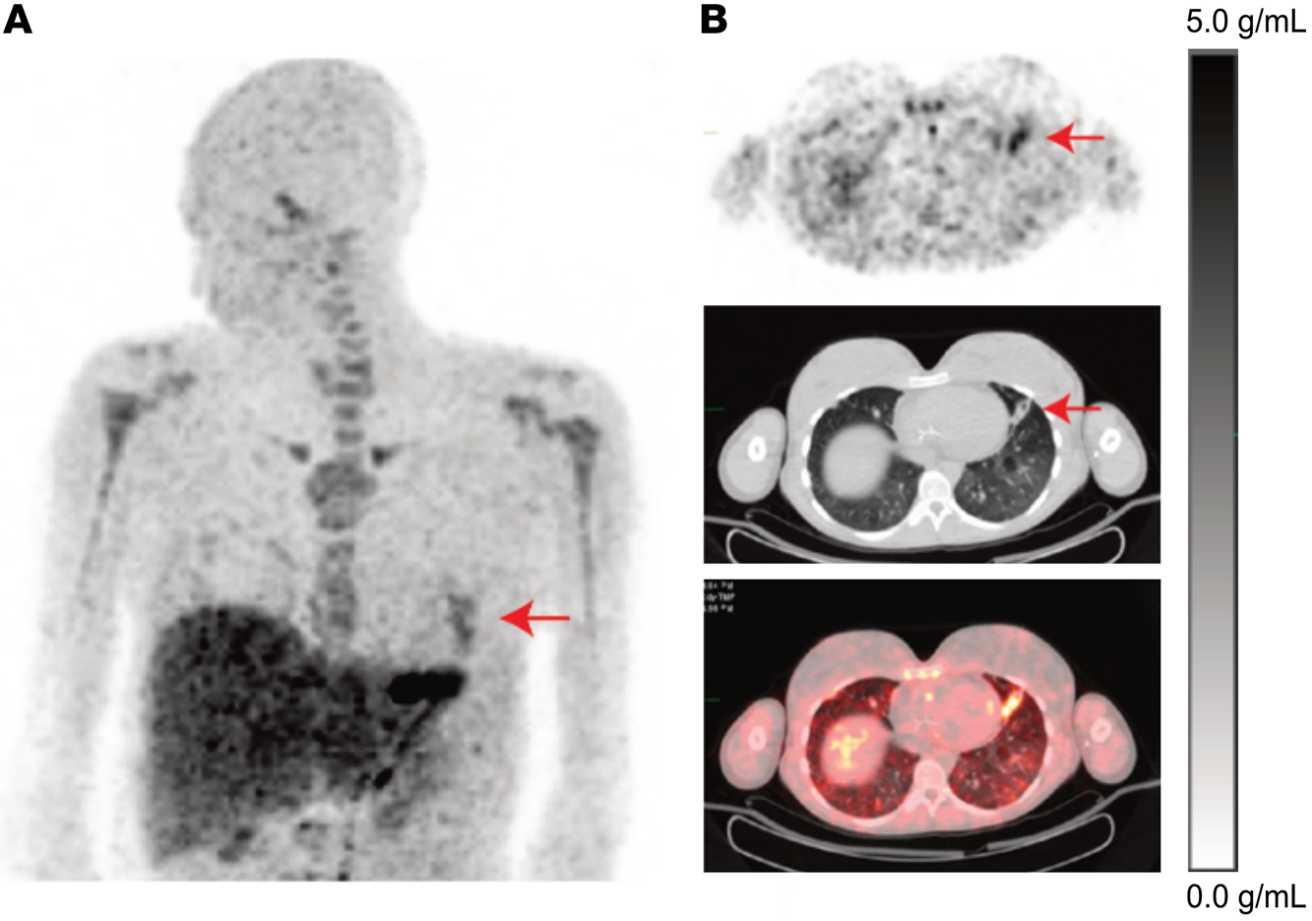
Acute exacerbation of cystic fibrosis. (A) A 64-year-old man with known lung adenocarcinoma underwent a [^18^F]-FDG (549 MBq) and then a [^11^C]-TMP (563 MBq) PET/CT 2 days later. The [^18^F]-FDG image was acquired starting 71 minutes after injections. Whole-body maximum intensity projection (MIP) images demonstrate the difference in biodistribution of the tracers. In the lungs, [^18^F]-FDG is taken up both by metabolically active tumor and inflammatory cells, whereas [^11^C]-TMP is not. (B) A comparison MIP image of a 44-year-old woman with cystic fibrosis and chronic lung infections, who underwent a [^11^C]-TMP PET/CT (780 MBq). The image was acquired starting 78 minutes after injection. The PET images show several foci of infection in the chest (red arrows). Other sites of signal include the liver, the kidneys, red bone marrow, and the stomach. PET images are scalled 0-7 g/mL SUV.
